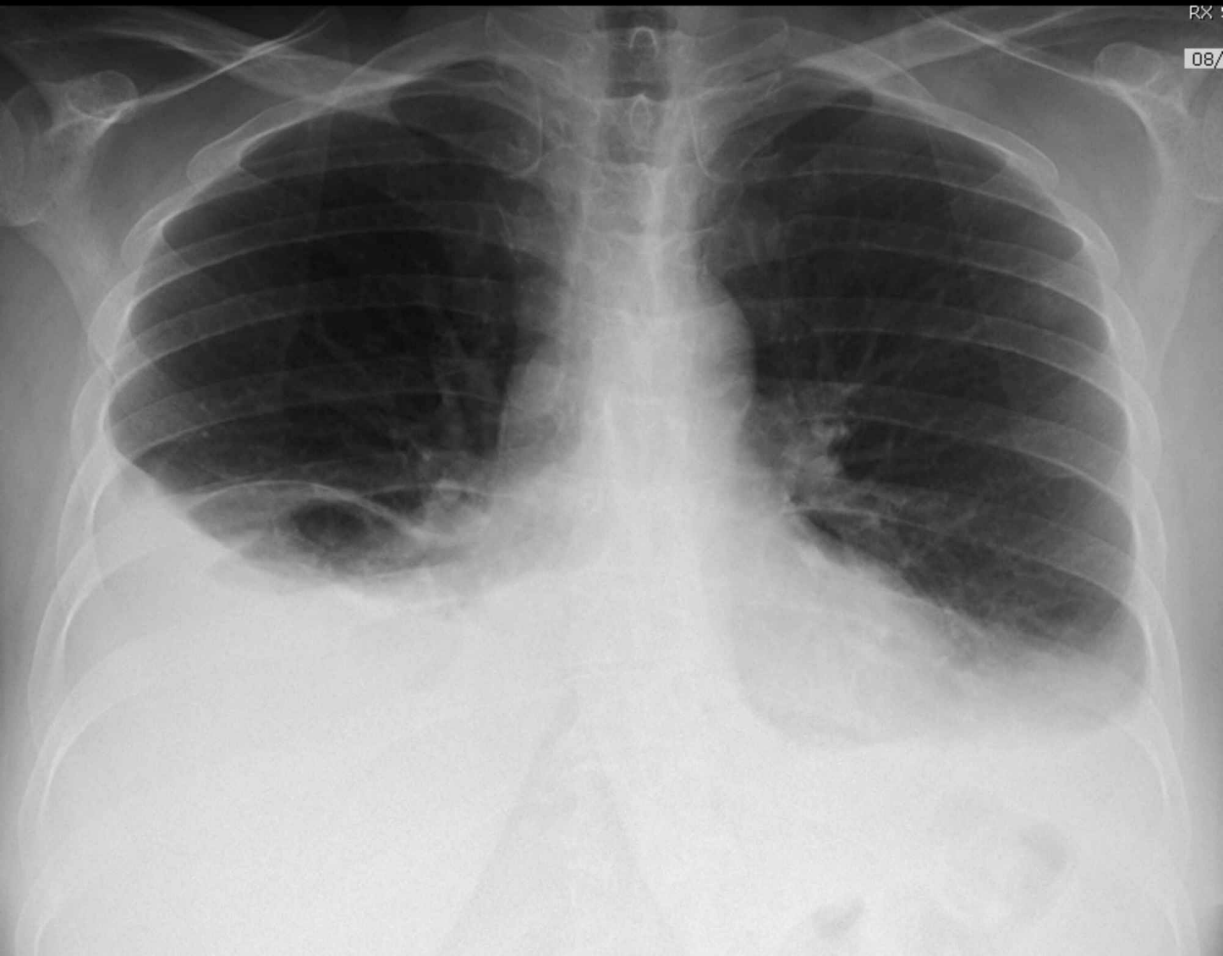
Chest X-ray. Bilateral pleural effusion distributed in a typical fashion, larger on the right side.
